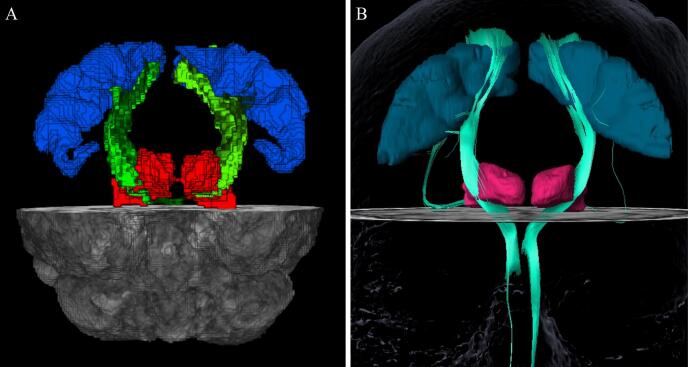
Tractography Methods. Three-Dimensional tractography results from a single patient in T1-weighted space demonstrating streamlines (green) between the thalamus (red) and precentral gyrus (blue) using the probabilistic (A) and deterministic methods (B). Three-dimensional reconstructions of probabilistic and deterministic fiber tracts were created using MRtrix (https://www.mrtrix.org) and Brainlab, respectively. (For interpretation of the references to color in this figure legend, the reader is referred to the web version of this article.)
